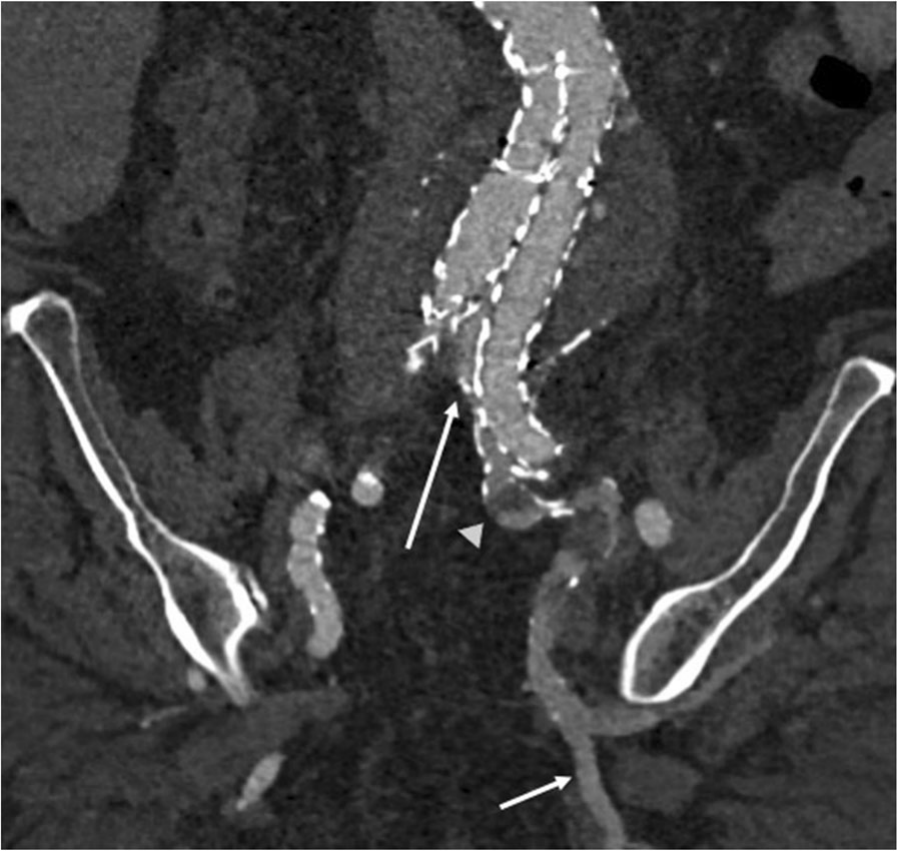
Reformatted CT angiogram image showing contrast filling of left internal iliac artery (arrowhead) and type II endoleak in the aneurysm sac (short arrow). The anterior division of the left IIA is demonstrated with the long arrow
